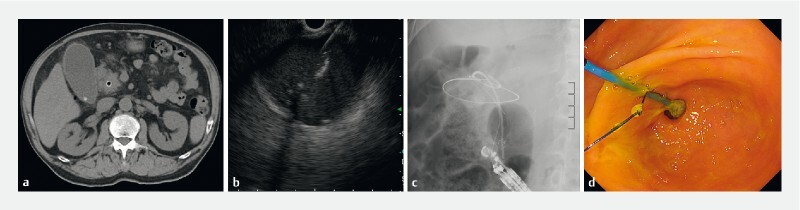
Usefulness of modified single‐pigtail plastic stent inserted into a fully covered self-expandable metal stent (FCSEMS) in endoscopic ultrasound-guided gallbladder drainage.
